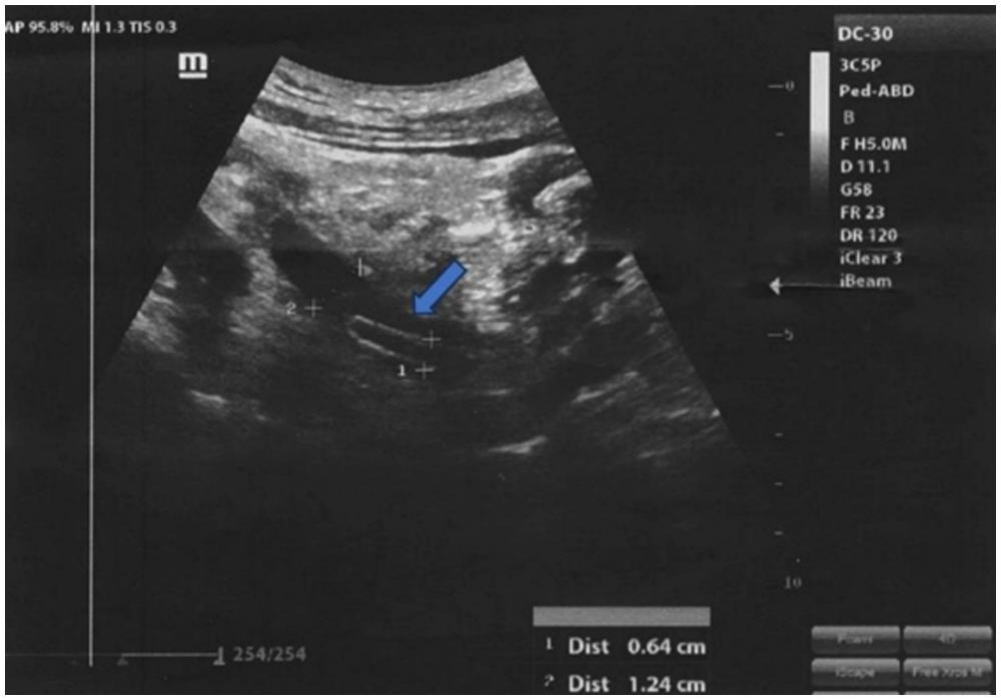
Renal ultrasound of the right kidney showed a cylindrical structure measuring 6 mm in diameter and 6 cm in length in the distal part of the right ureter.
